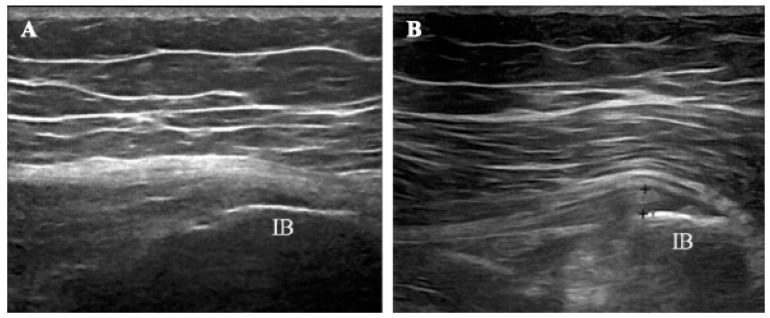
Longitudinal views of the gluteus medius tendon from a patient in the study before (A) and after (B) combined ESWT. At baseline, the ultrasound image showed hypoechoic thickening at the tendon’s insertion onto the iliac bone (IB). Irregularities of the tendon’s superior contour were also evident. In contrast, a marked improvement in the morphological aspect of the tendon was evident at T2, as shown by the more homogeneous tendon fibrillar pattern and reduced thickness.
